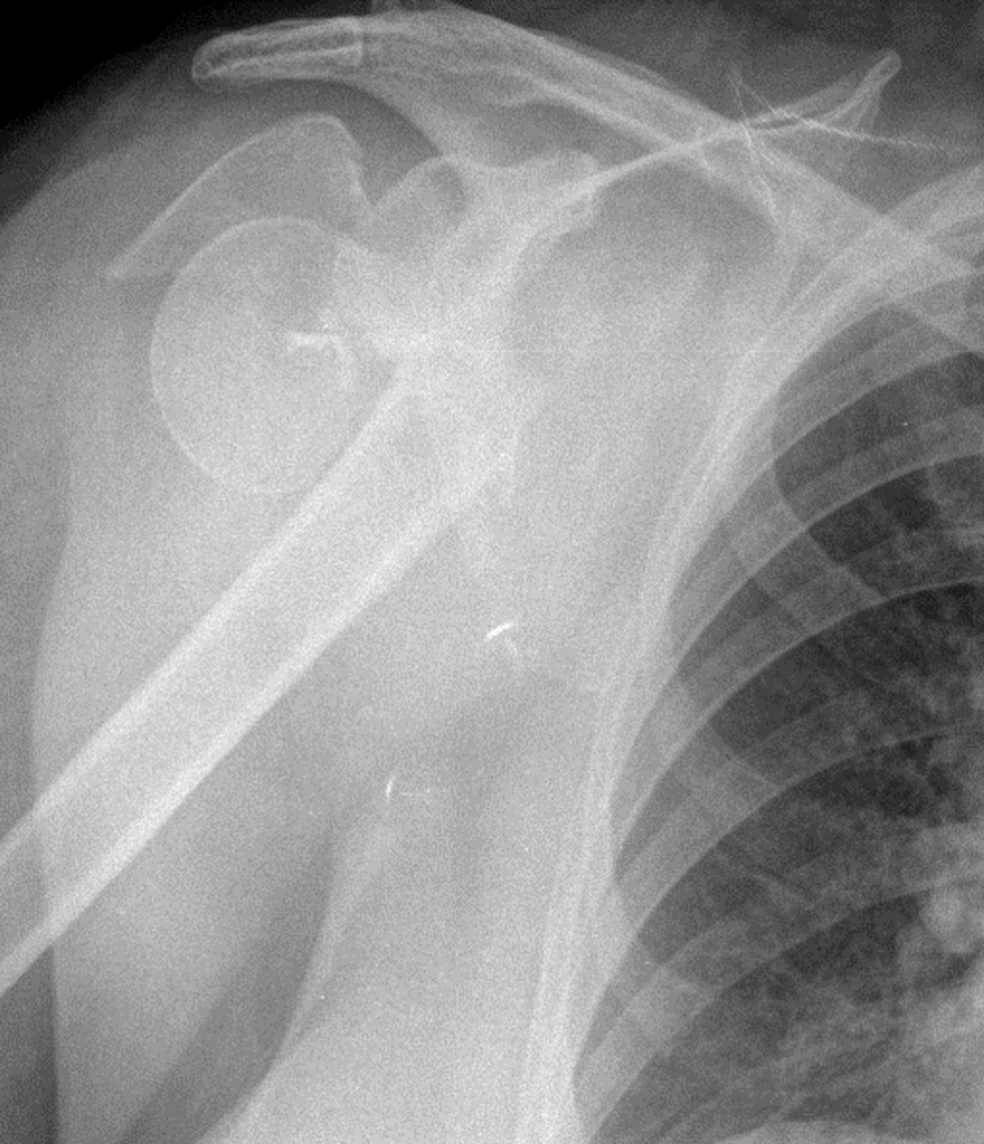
A 75-year-old female’s preoperative X-ray.
